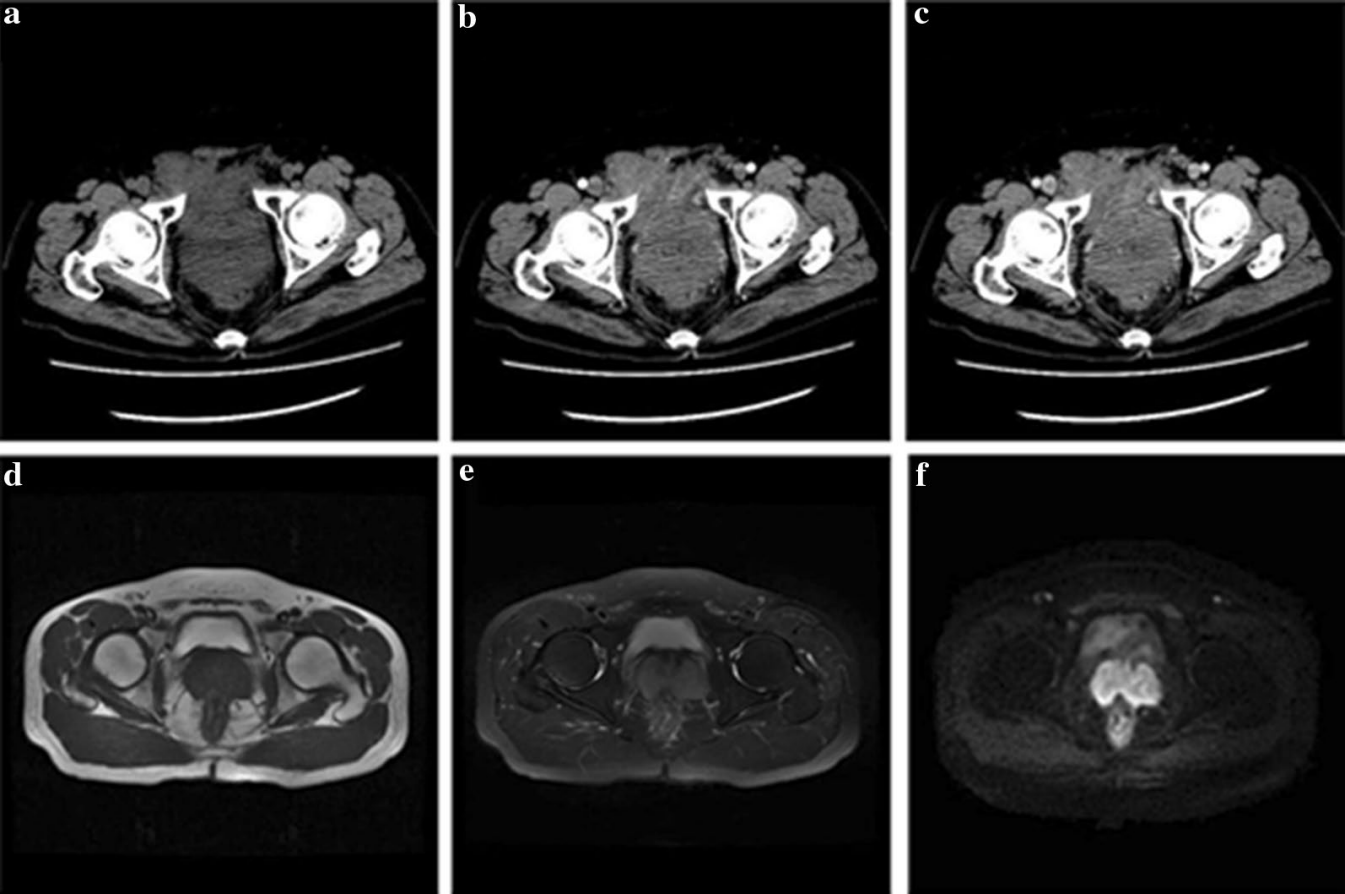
Preoperative diagnosis was performed by pelvic CT or MRI examination. The results suggested that the prostate was significantly augmented, the internal density of the prostate was uneven and spot-like calcified. Pelvic CT figure: a plane scan, b curve arterial phase, c curve vein; MRI figure: d T1W1, e T2W1, f DWI
